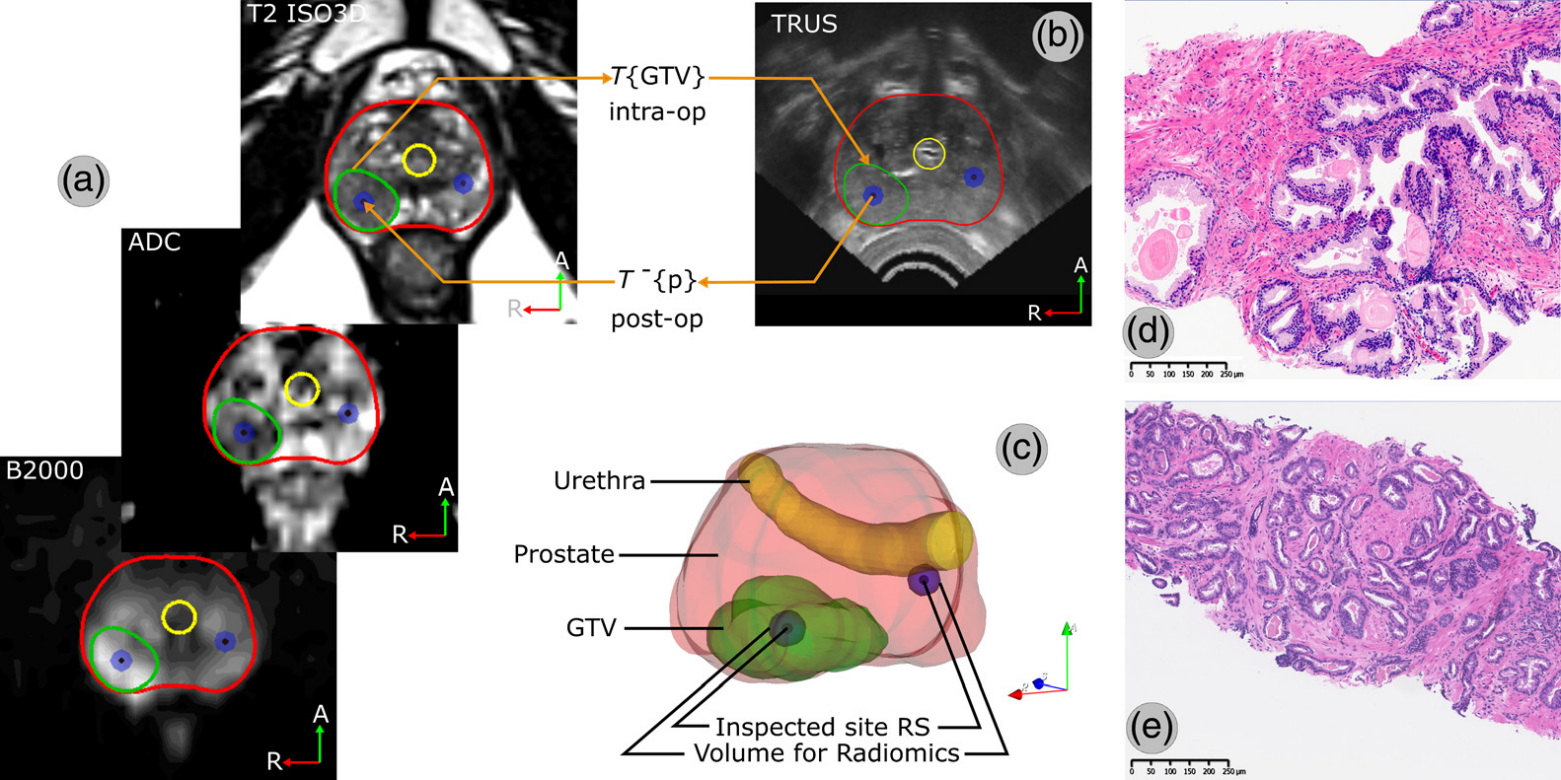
Sample image registration and co-location results. (a) GTV originally segmented on preoperative mpMRI is (b) projected over the real-time TRUS using a surface-based elastic registration algorithm; the inverse process allows identifying the MRI coordinates corresponding to the inspected sites. (c) A 3D model of the plan can be rendered. (d) Pathological image samples of benign prostatic parenchyma and (e) acinar adenocarcinoma GS: 3+4=7, ISUP GG=2, and HG: 40% to 50%.
